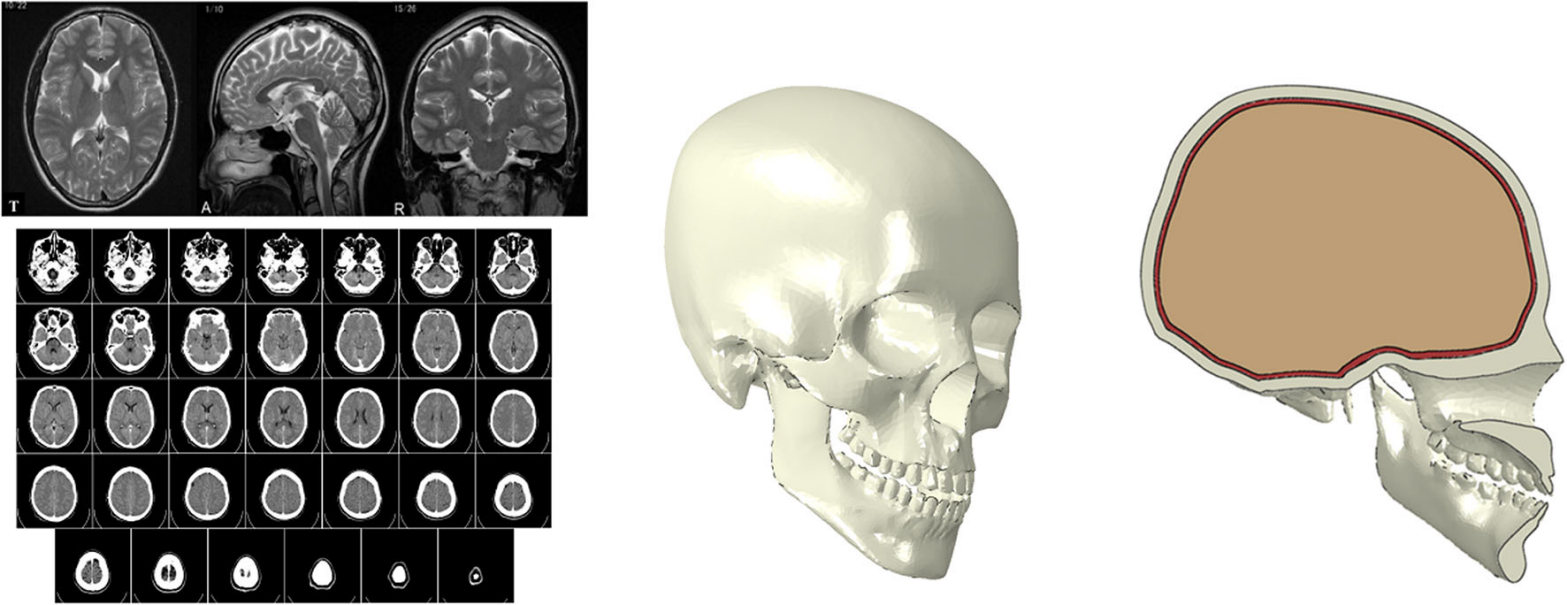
Three-dimensional geometric models of the brain and skull created using computed tomography images
